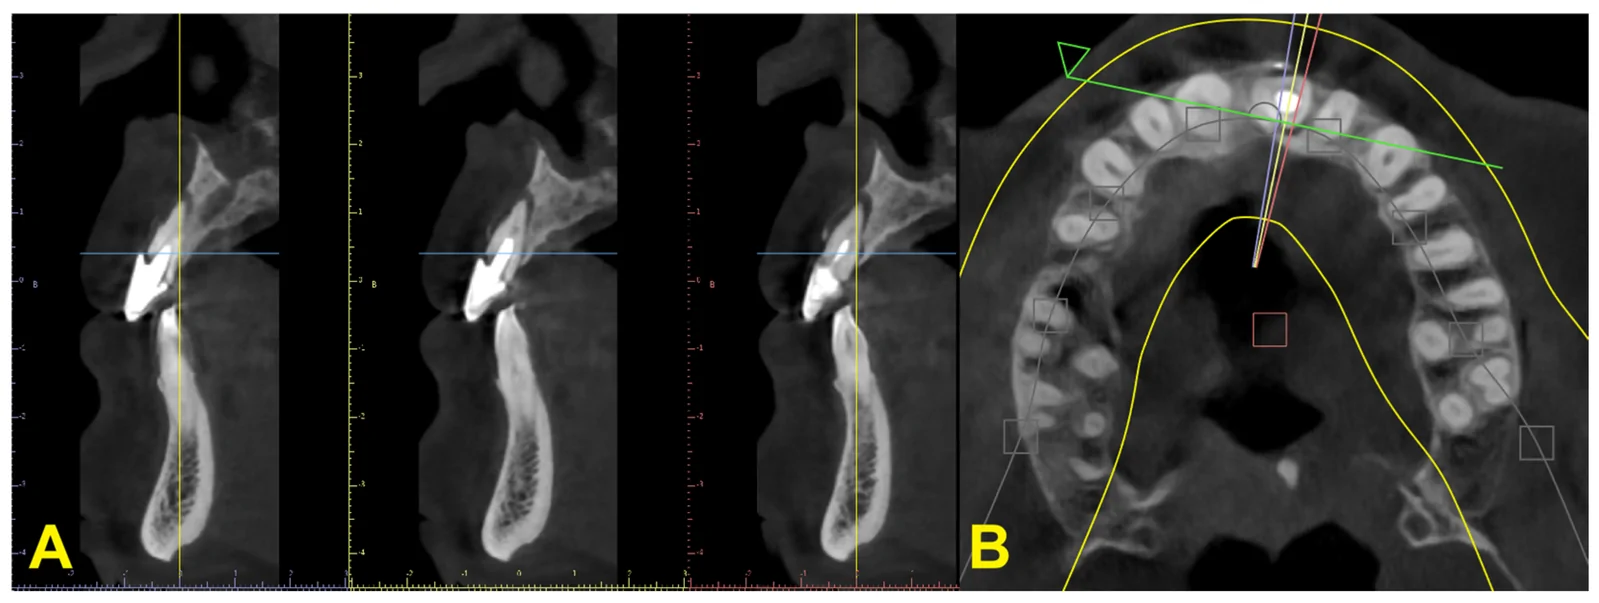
Cone-beam computed tomography of tooth 21 before extraction: (A) cross-sectional views; (B) axial view.
